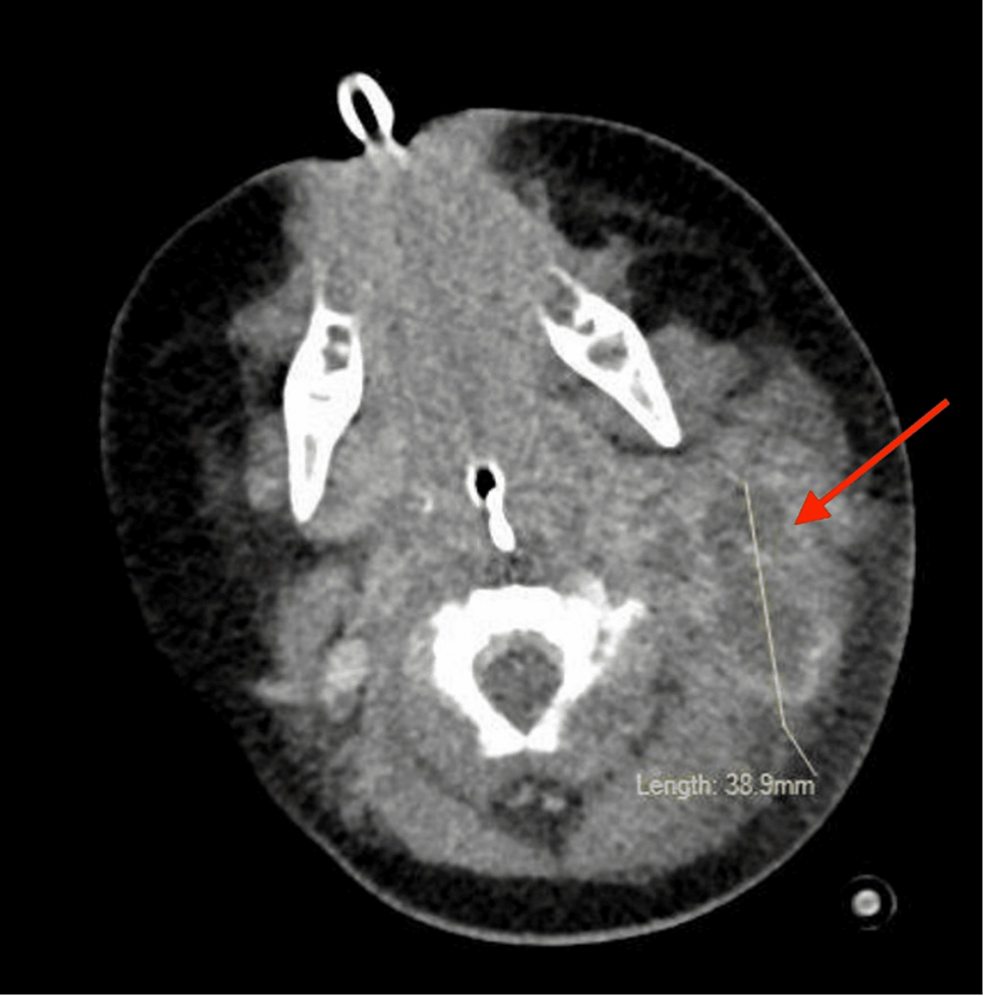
CT of the soft tissue of the neck with contrast Loculated thick-walled centrally hypodense collection within the left upper jugular chain measuring 38.9 mm that extends deep into the left sternocleidomastoid (arrow) CT: computed tomography
